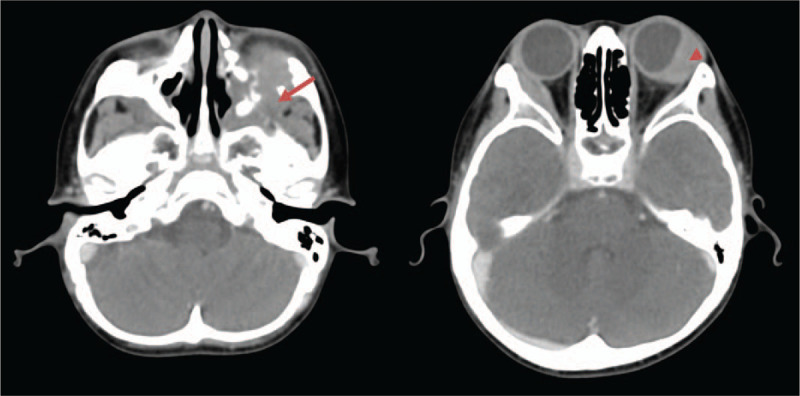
Computed tomography of the orbit demonstrated an enhancing soft tissue mass with extra-ocular muscle involvement of the left orbit (arrow head) and bone destruction in the left maxillary sinus (arrow).
